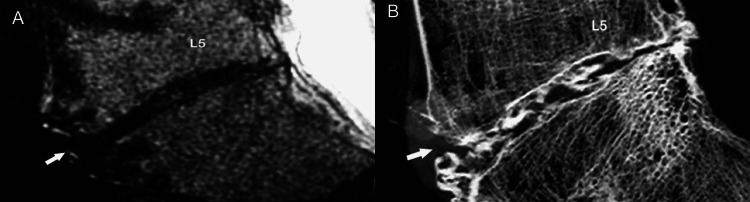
Disc calcification. (A) Sagittal T2-weighted MR image shows predominant low signal intensity of the intervertebral disc (arrow) at the markedly narrowed L5-S1 interspace. (B) Corresponding low-kilovoltage contact radiograph reveals densely calcified disc (arrow) at that level. There is loss of disc height, eburnation of adjacent bone, and spur formation.
